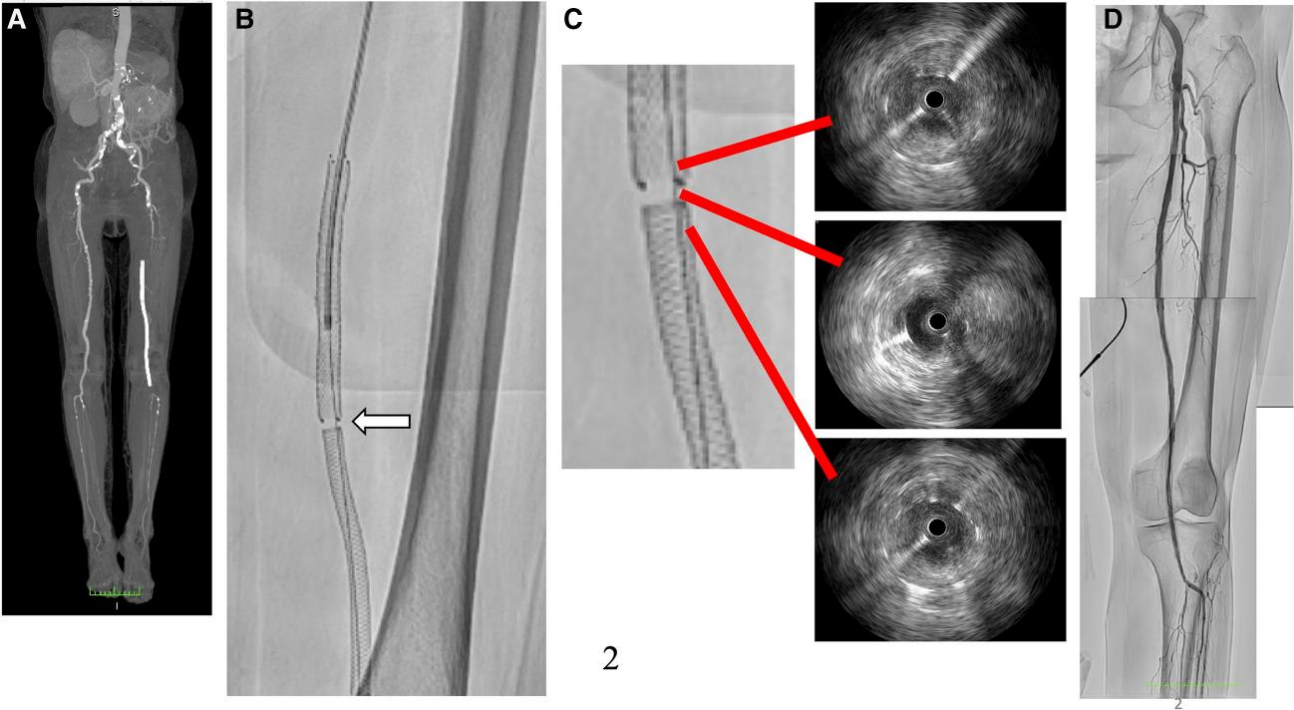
Computed tomography angiogram showed total occlusion from ostial superficial femoral artery to popliteal artery (*A*). The angiogram revealed a gap (white arrow) between the stents that were previously implanted (*B*). According to the intravascular ultrasound results, there was a significant amount of thrombus in the stents and evidence of neointimal hyperplasia in the gap (*C*). Successful revascularization of the superficial femoral artery to popliteal artery (*D*).
